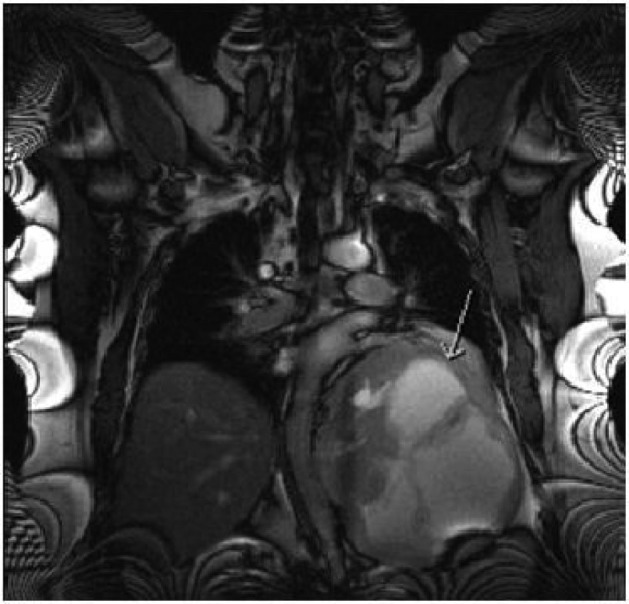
MRI features of the mass (arrow).
